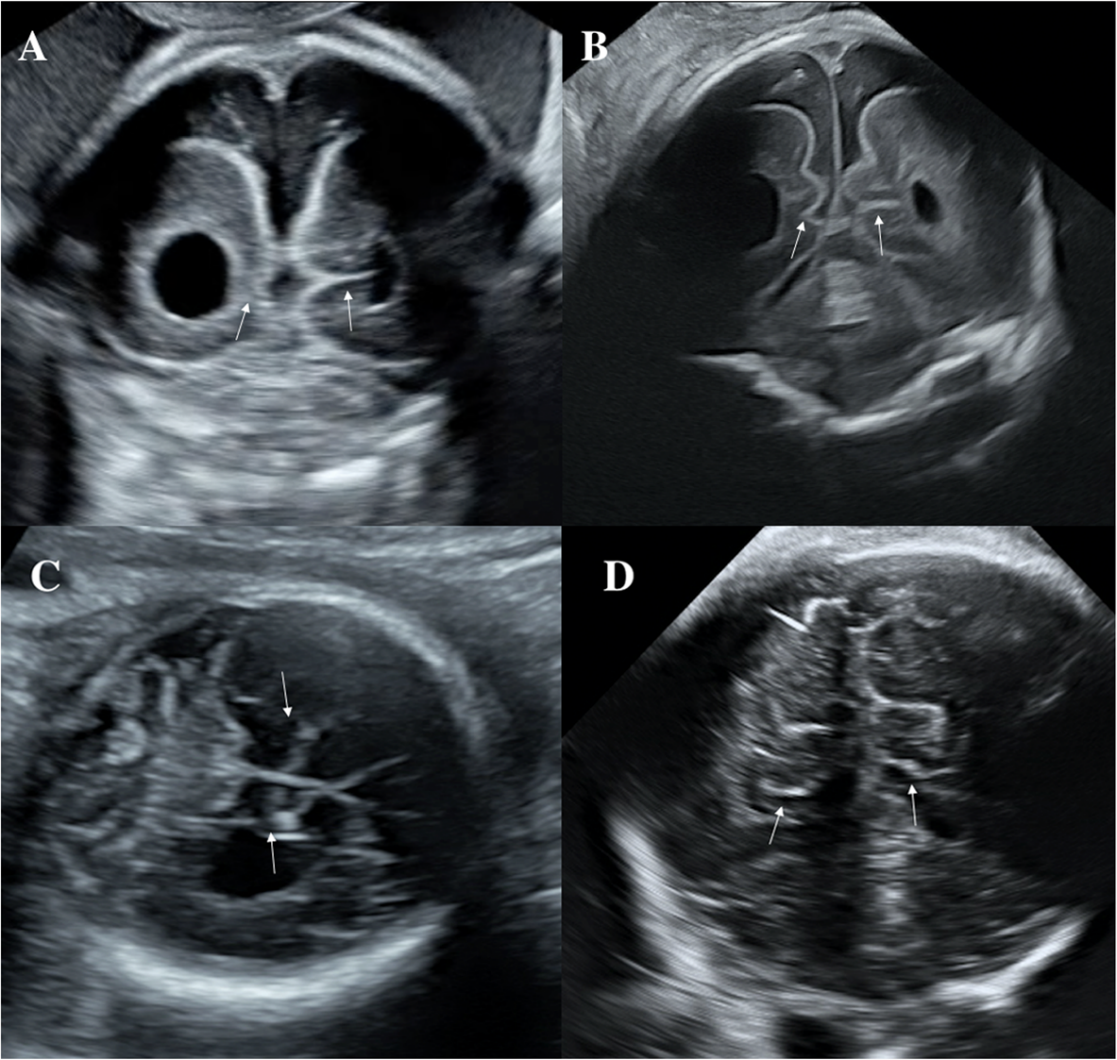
Asymmetry in the development and ‘catch-up growth’ of calcarine sulcus in a IMVM fetus with unilateral ventriculomegaly. Ultrasound image of calcarine sulcus on coronal transcerebellar plane at 25 weeks (a), 27 weeks (b), 30 weeks (c) and 32 weeks (d) of gestation. a the calcarine sulcus was grade 1 on one side and was grade 2 on the other side. b the calcarine sulcus was grade 2 on one side and was grade 4 on the other side. c the calcarine sulcus was grade 4 on both sides. d the calcarine sulcus was grade 5 on both sides
